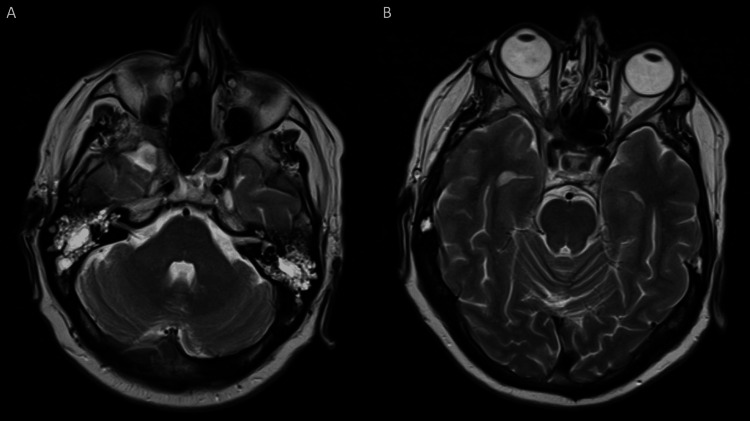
Follow-up MRI of the brain with contrast. Axial T2-weighted MRI images (A) at the level of the posterior cranial fossa and (B) at the level of sella turcica. MRI of the brain with contrast performed four months after the initiation of adjuvant chemotherapy demonstrates further reduction in the size of the residual tumour compared to the MRI obtained four months earlier.
